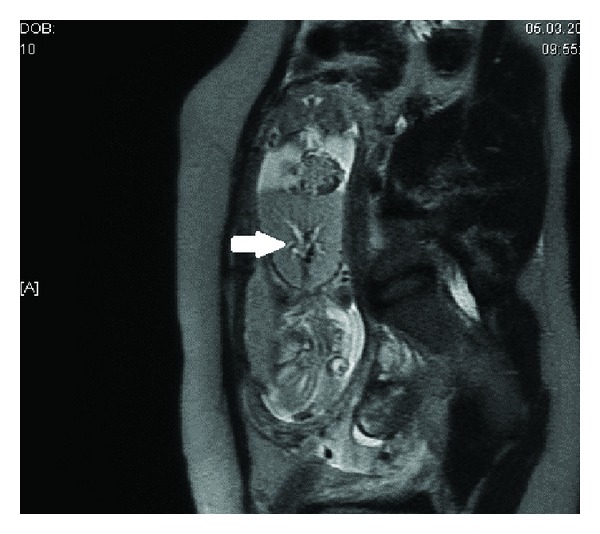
Antenatal MRI of case 1 showing dilated airways (arrow), hyperxpanded lungs, inverted diaphragm, and ascites.
